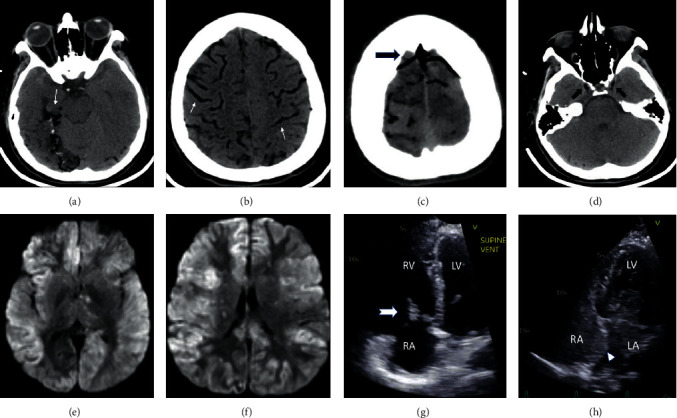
(a, b) Computerized tomography (CT) of the brain axial sections showing air embolism in the cortical branches of the posterior cerebral, middle, and anterior cerebral arteries, respectively (white arrows). (c) Same CT scan showing air in the superior sagittal sinus and cortical veins (black arrow). (d) CT scan showing air in the bilateral cavernous sinus (black arrow). (e, f) Diffusion-weighted sequence of a magnetic resonance image of the brain showing diffuse bilateral cortical anoxic injury. (g, h) TTE showing tricuspid valve vegetation (white arrow) and location of interatrial septum and PFO, respectively (white arrowhead). Bubbles in the left atrium and ventricle that crossed within 3 cardiac cycles.
